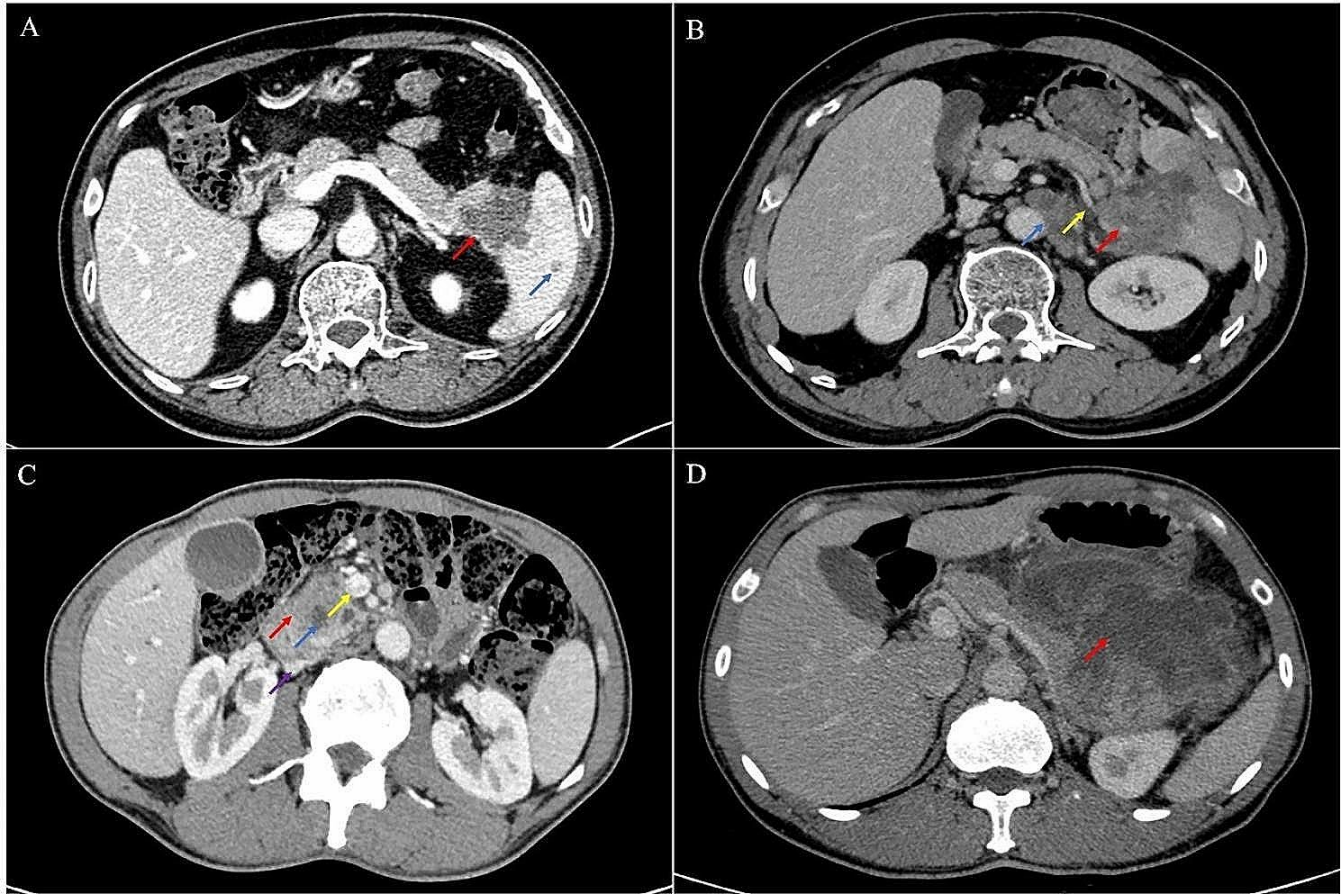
Contrast-enhanced CT scan of the abdomen. A. A low density tumor of the pancreatic tail (red arrow) with splenic metastasis (blue arrow). B. A tumor located in the tail of the pancreas with ill-defined margins and heterogenous enhancement (red arrow). The splenic artery was encased by the tumor (yellow arrow). Enlarged lymph nodes were seen in the left renal portal and paraaortic (blue arrow). C. A slightly low-density tumor with heterogenous enhancement was seen on the head of the pancreas (red arrow), and lower density nodule was seen within the tumor (blue arrow). The tumor compressed the inferior vena cava (purple arrow) and involved the SMV (yellow arrow). D. A large tumor originating from the tail of the pancreas. The tumor compresses the tail of the pancreatic body and involves the left kidney. Intratumoral bleeding was observed (red arrow)
